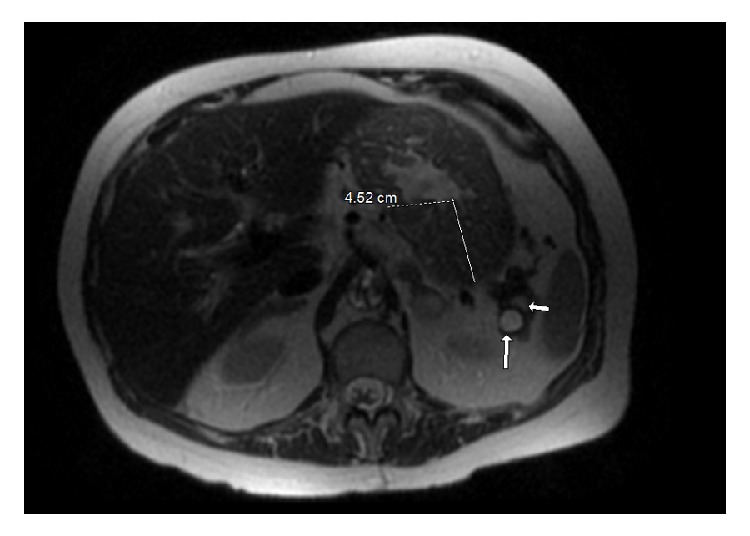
MRI abdomen showing diffuse gastric wall thickening (4.52 cm) with a small pancreatic cystic lesion (arrows).
